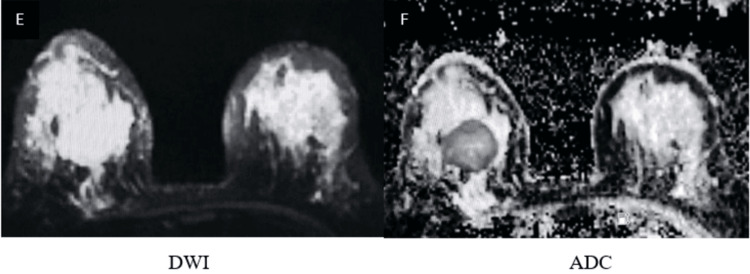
Round to oval shape lesion in the right breast demonstrates marked heterogenous peripheral diffusion restriction on DWI (E, F) DWI: diffusion-weighted imaging; ADC: apparent diffusion
